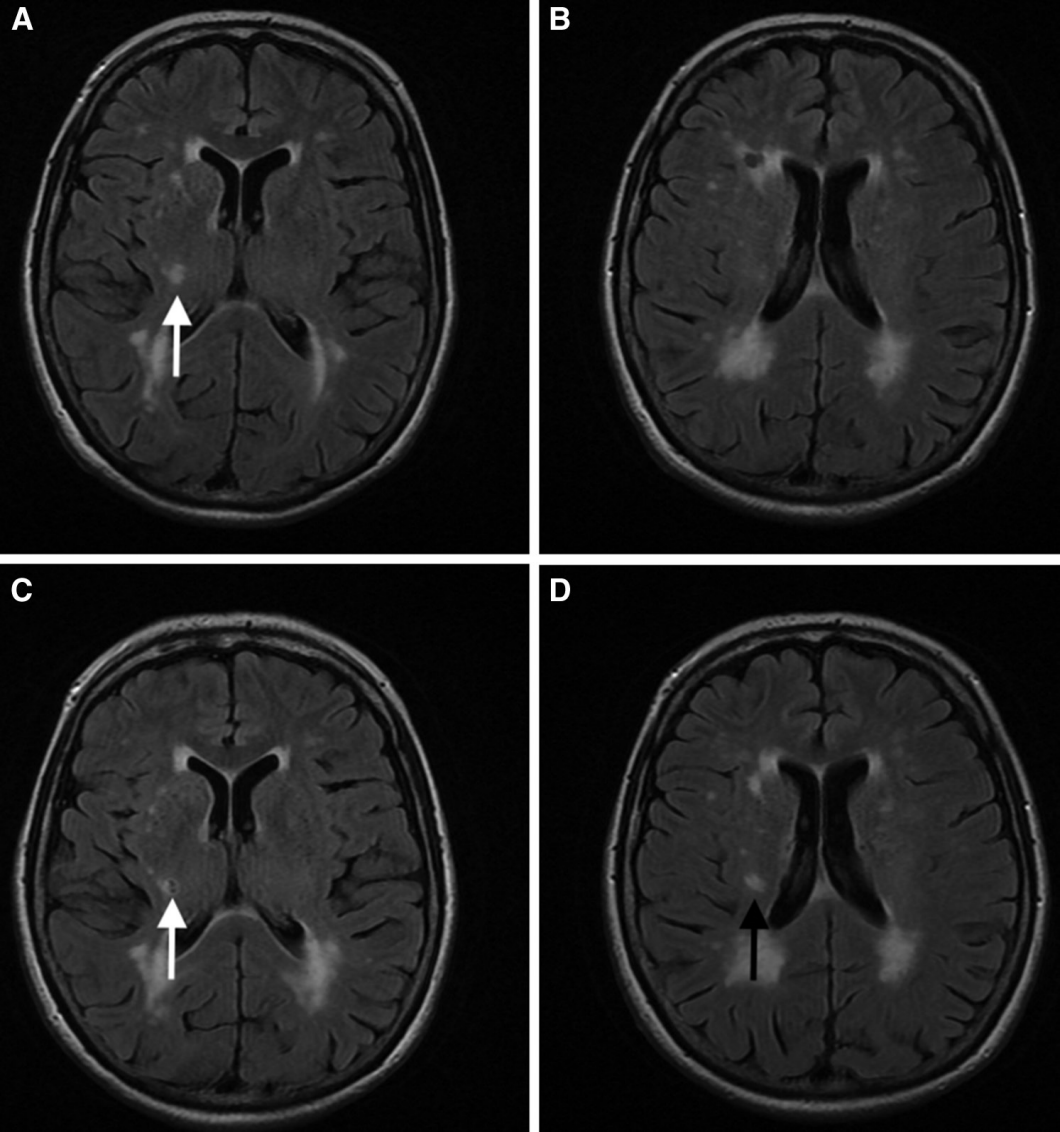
The occurrence of a white matter hyperintensity (WMH) cap adjacent to index lacunar lesion. A, Baseline magnetic resonance imaging (MRI), performed 1 d after stroke onset, sporadic symptomatic lacunar infarct in the right internal capsule (white arrow; fluid-attenuated inversion recovery [FLAIR]). B, Baseline FLAIR image on MRI slice superior to the index lesion. C, Follow-up MRI at 1 y (353 d) after index stroke, partial cavitated lesion (lacey-like appearance) on FLAIR (white arrow). D, Follow-up FLAIR image on MRI slice superior to the cavitated index lesion, showing a WMH cap adjacent to the index lacunar lesion (black arrow).
